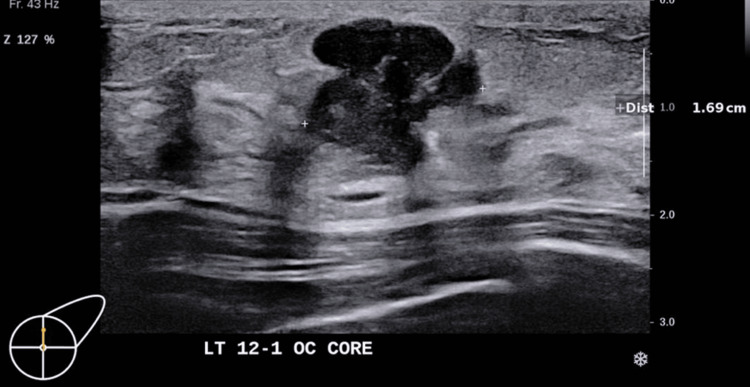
Ultrasound left breast of patient A.
